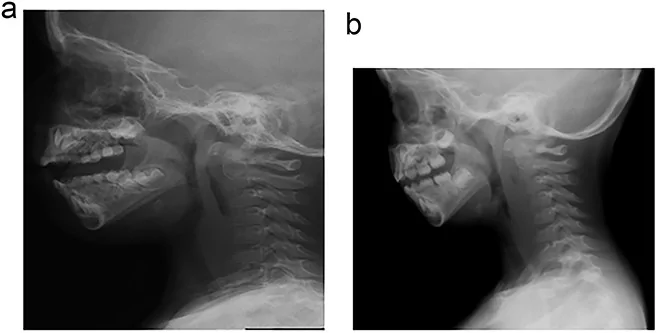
Lateral soft tissue neck X-rays at Day 5 (a) and Day 9 (b) of intravenous antibiotic course showing progressive resolution of retropharyngeal air foci.
